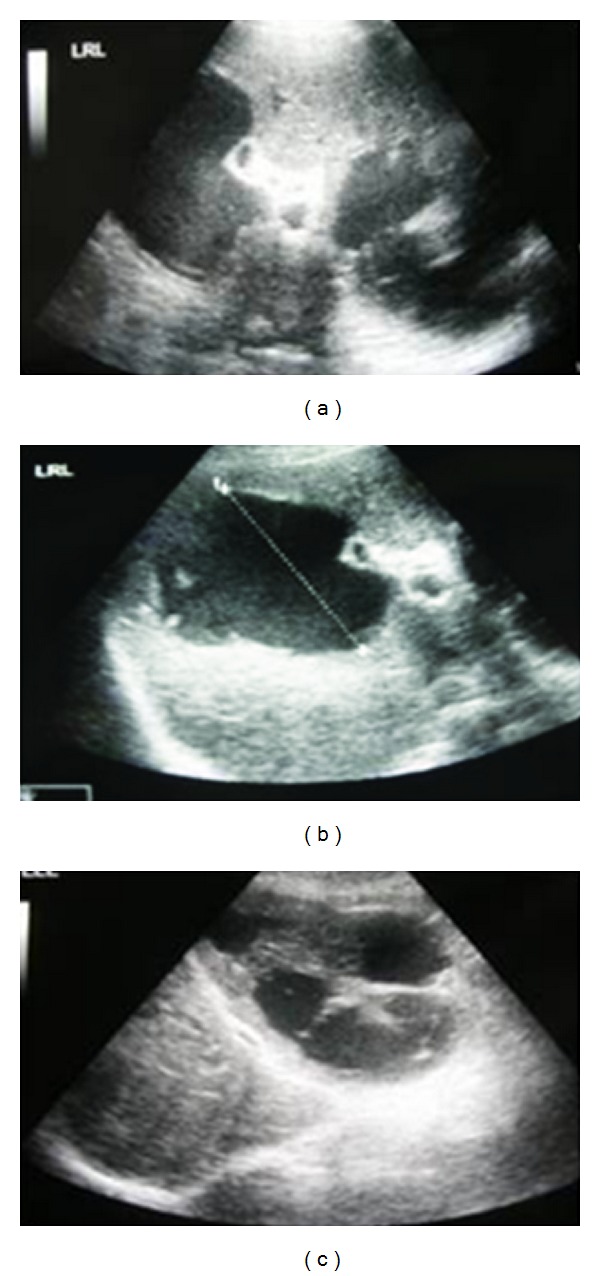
Abdominal ultrasound demonstrating cystic lesions in the right lobe of the liver that also involves almost the entire left liver lobe.
